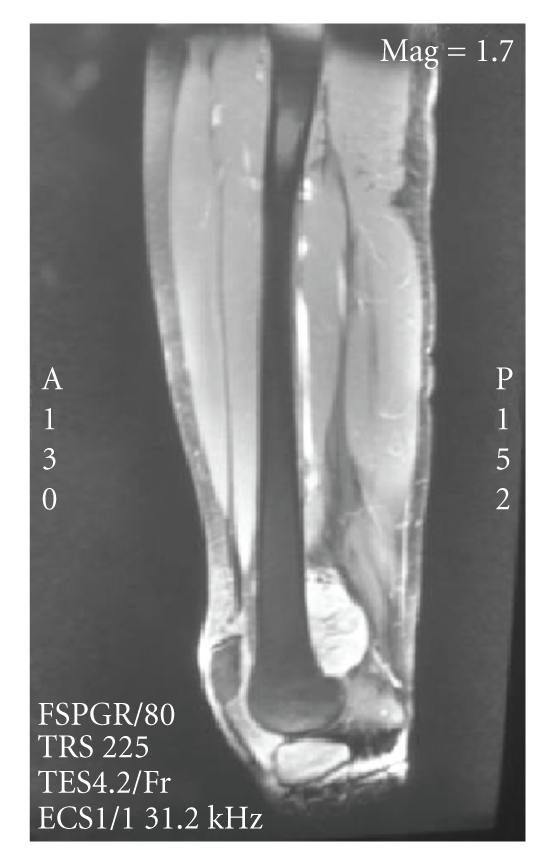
Preoperative MRI showed a relatively dense and well-demarcated ossified mass at a juxtacortical
